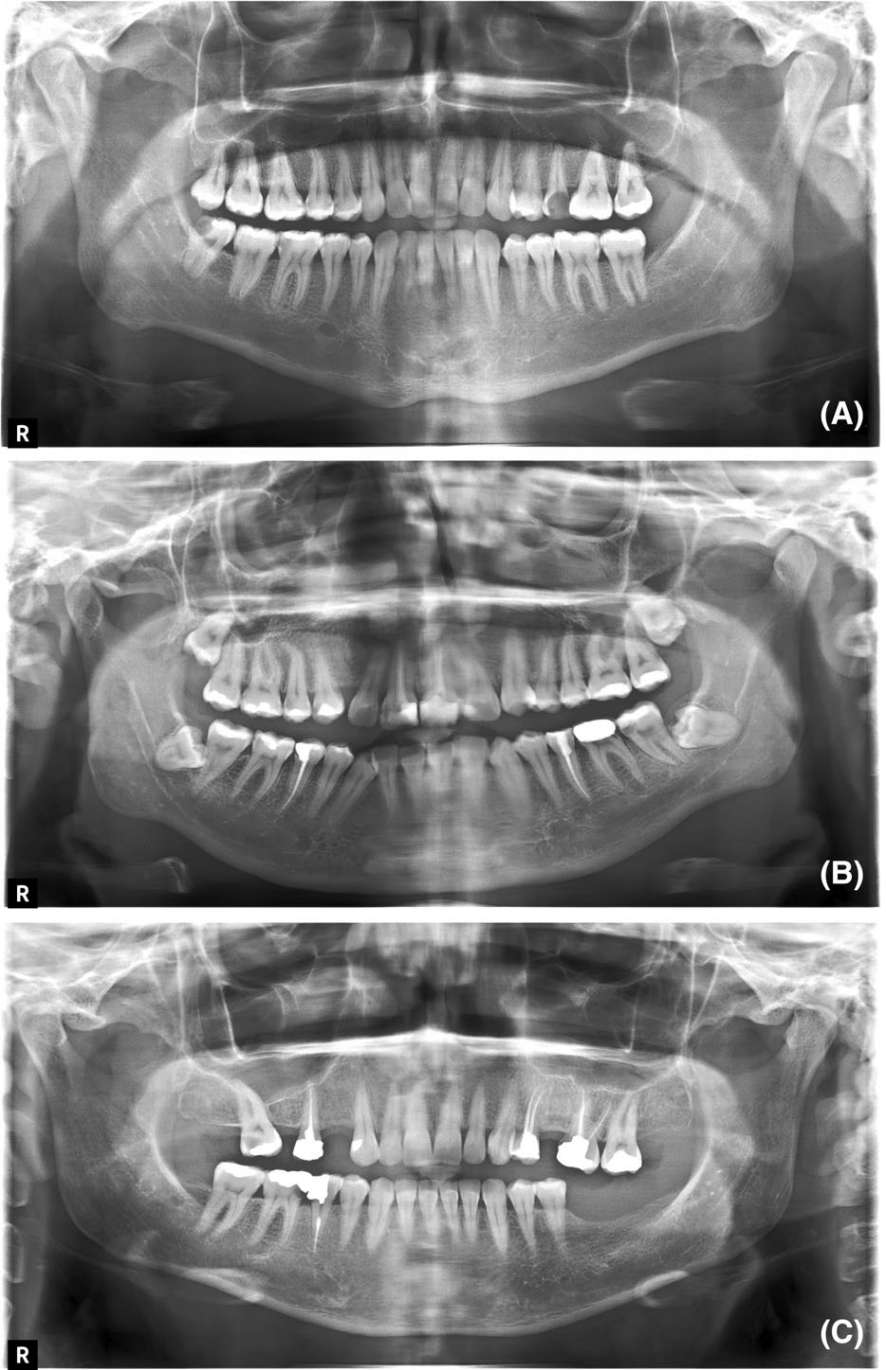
Examples of radiographic images: (A) An unacceptable radiographic image classified in this study based on Palatoglossal air space shadow error which can obscure critical anatomical details, potentially leading to misdiagnosis or the need for additional imaging procedures. (B) An acceptable radiographic image classified in this study based on Palatoglossal air space shadow error. (C) An excellent radiographic image classified in this study based on Palatoglossal air space shadow error.
